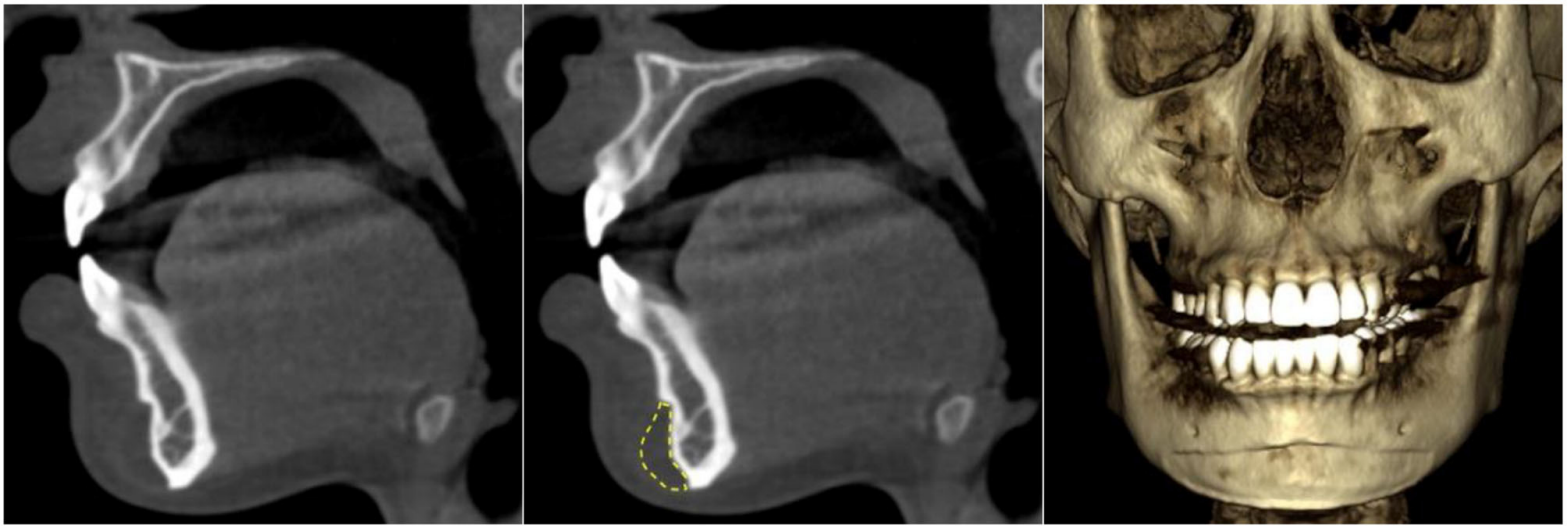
Mandibular bone resorption on the labial surface caused by a chin implant observed with cone-beam CT. The resorption was obvious in both sagittal view and volume rendering. The chin implant is circled yellow.
